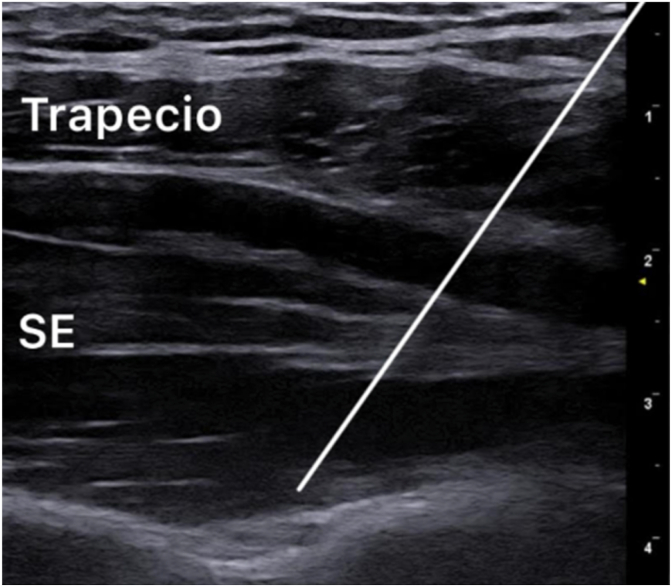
Ultrasound image of the suprascapular notch showing the trapezius and supraspinatus (SS) muscles, and needle trajectory toward the suprascapular nerve. Authors' own images.
